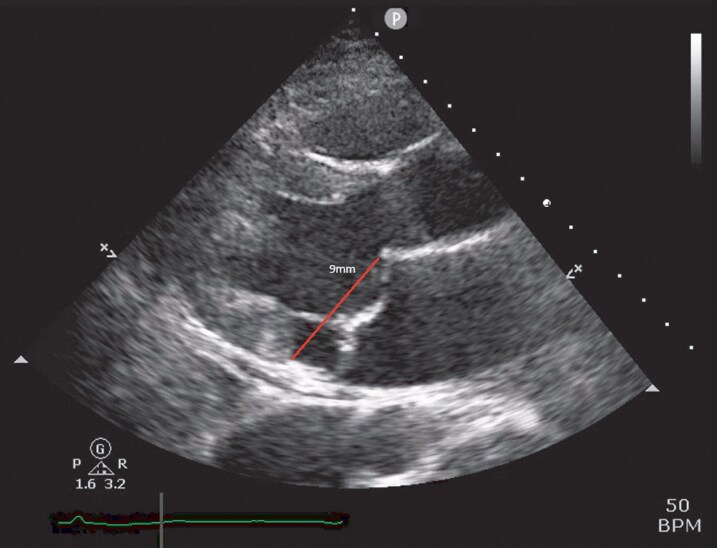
Parasternal long-axis view in mid-systole showing mitral annular dysjunction distance of 9 mm.
